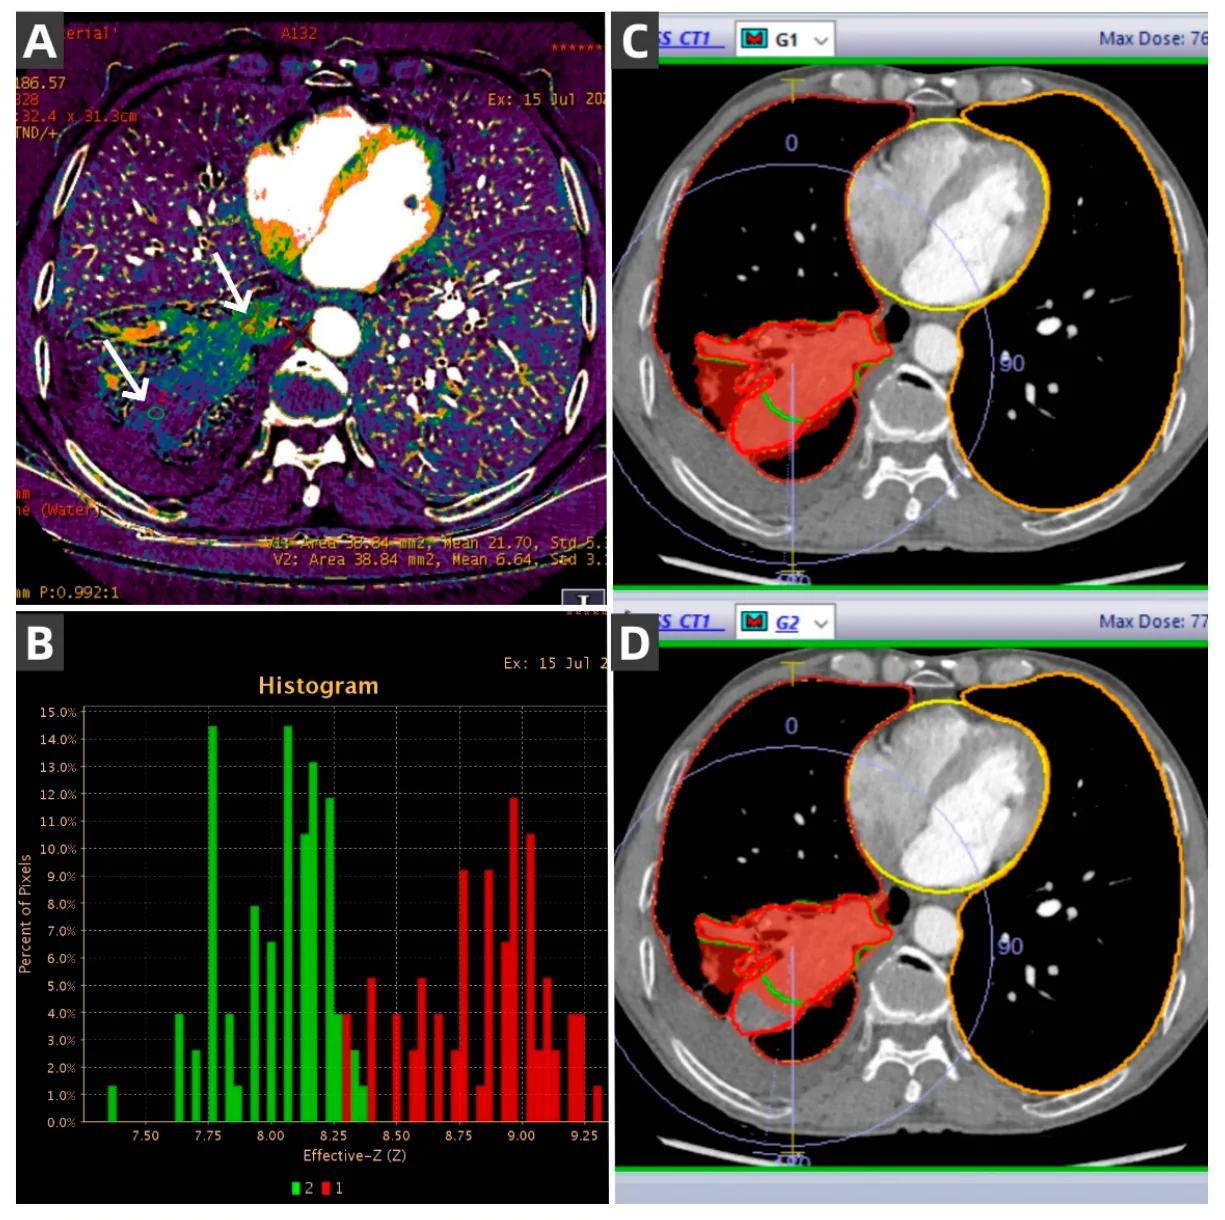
Iodine map-based delineation of radiotherapy target volume reduces inclusion of necrotic or atelectatic regions compared with conventional CT. (A) Two regions of interest (ROIs) are delineated on the iodine density map (white arrows): ROI 1 (red) shows high iodine concentration, indicating viable tumor tissue; ROI 2 (green) shows low iodine concentration, indicating necrosis or atelectasis. (B) Effective atomic number (Z_eff_) values corresponding to the two ROIs, demonstrating that the Z_eff_ value of the necrosis or atelectasis region (green) is significantly lower than that of the tumor region (red). (C) Radiotherapy target volume (red shaded area) delineated based on conventional contrast-enhanced CT. (D) Iodine map-based target volume delineation (red shaded area), which excludes necrosis or atelectatic regions and results in a substantially smaller volume, thereby potentially avoiding unnecessary irradiation of non-viable atelectatic lung tissue.
